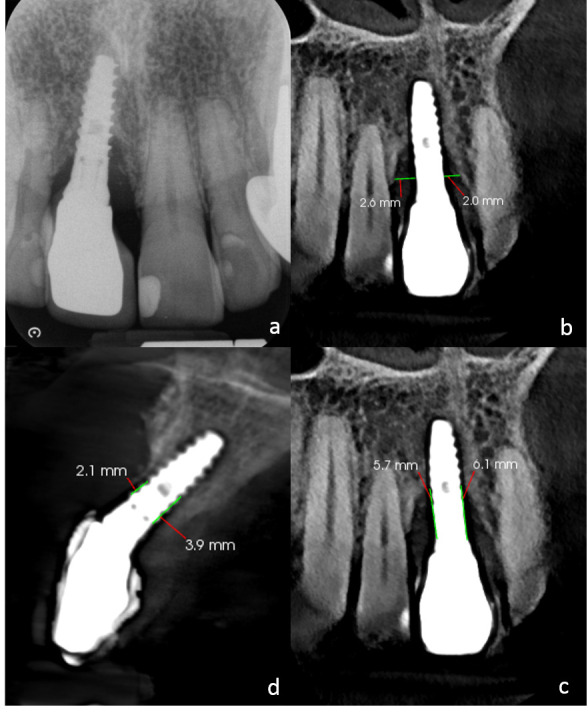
(a) Radiografía periapical digital donde se observa defecto vertical en mesial y distal del implante. Reconstrucciones multiplanares de tomografía computarizada de haz cónico con aplicación de algoritmo para la reducción de artefacto, que muestran en cortes coronales (b y c), las mediciones obtenidas de la profundidad y ancho del defecto óseo en mesial y distal del implante, en el corte sagital (d), se evidencia el compromiso de la tabla ósea vestibular (dehiscencia).
